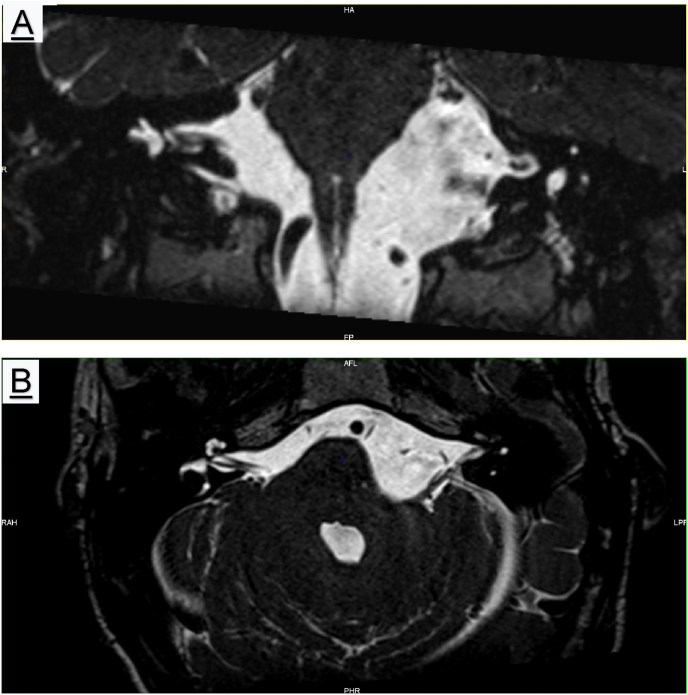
A: Postoperative MRI T2 coronal; B: Postoperative MRI T2 axial showing decompression of the nerve.
